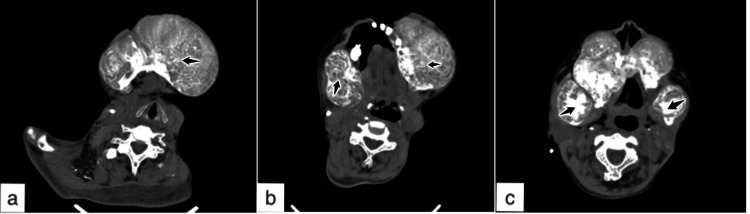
Contrast-enhanced CT face and neck (axial views). (a-c) Expansile lesions involving mandible and maxilla with internal calcified component.
